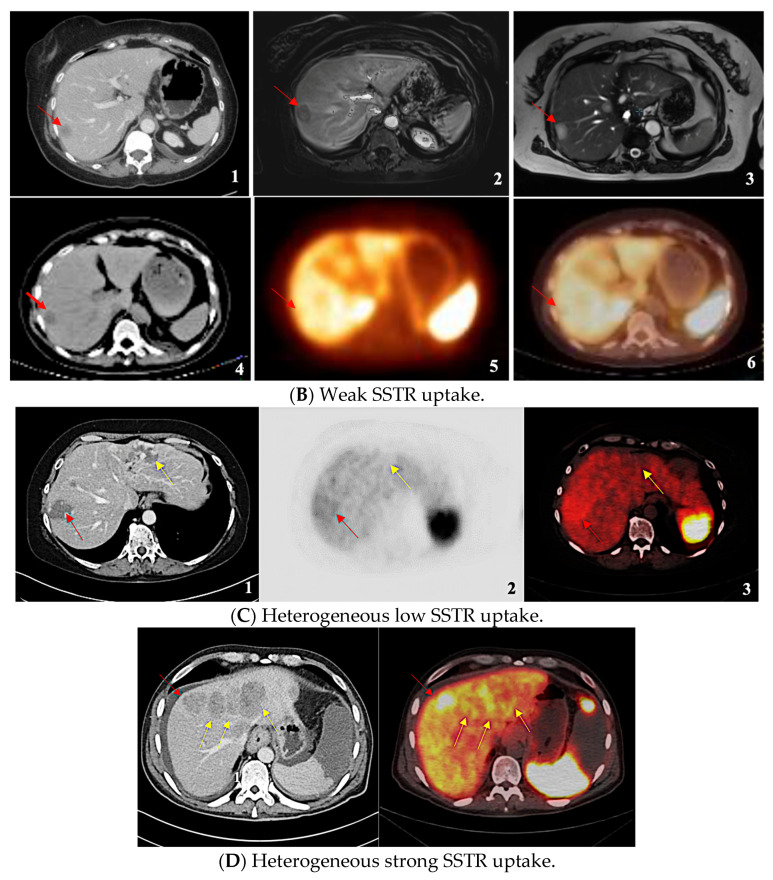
Different SSTR uptake patterns in study population: PET/CT and CT/MRI image comparison. Part (A) shows three SSTR negative metastatic liver lesions by different imaging methods: (1) basal CT scan acquired during [^68^Ga]Ga-DOTATATE PET/CT (axial); (2) [^68^Ga]Ga-DOTATATE PET/CT (axial); (3) Fused imaging [^68^Ga]Ga-DOTATATE PET/CT (axial). Part (B) shows one SSTR weak uptake metastatic liver lesion by different imaging methods (red arrow): (1) abdomen CT scan (axial, venous phase); (2) abdomen CE MRI (axial, T1); (3) abdomen CE MRI (axial, T2); (4) basal CT scan acquired during [^68^Ga]Ga-DOTATATE PET/CT (axial); (5) [^68^Ga]Ga-DOTATATE PET (axial); (6) fused imaging [^68^Ga]Ga-DOTATATE PET/CT (axial). Part (C) shows the coexistence of one SSTR weak and one SSTR absent uptake metastatic liver lesions by different imaging methods (red arrow for the weak lesion, yellow arrow for the absent): (1) CE CT scan (axial, venous phase); (2) [^68^Ga]Ga-DOTATATE PET/CT (axial, brightness and contrast have been edited by authors to better show metastatic lesions); (3) fused imaging [^68^Ga]Ga-DOTATATE PET/CT. Part (D) shows the coexistence of SSTR strong and SSTR absent/weak uptake metastatic liver lesions by different imaging methods (red arrows for the strong uptake liver lesion, yellow arrows for the absent/weak uptake liver metastasis): (1) CE CT scan (axial, venous phase); (2) fused imaging [^68^Ga]Ga-DOTATATE PET/CT (axial). SSTR = somatostatin receptor; PET/CT = Positron Emission Tomography/Computed Tomography; CT = Computed Tomography; MRI = Magnetic Resonance Imaging; ^68^Ga = Gallium-68; ^64^Cu = Cupper-64; CE = Contrast Enhanced.
